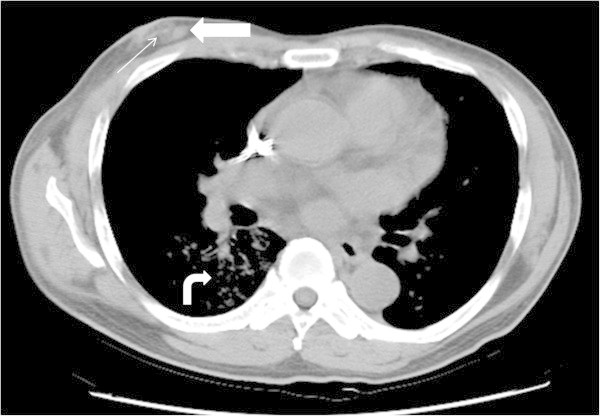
Breast panniculitis with vasculitis on axial non-contrast CT scan of chest. An oval nodule is located within the central inner region of the right breast (thick arrow) with increased infiltration of the subcutaneous adipose tissue (thin arrow). Pulmonary alveolar infiltrations within the right lower lobe of lung are also present (curved arrow).
